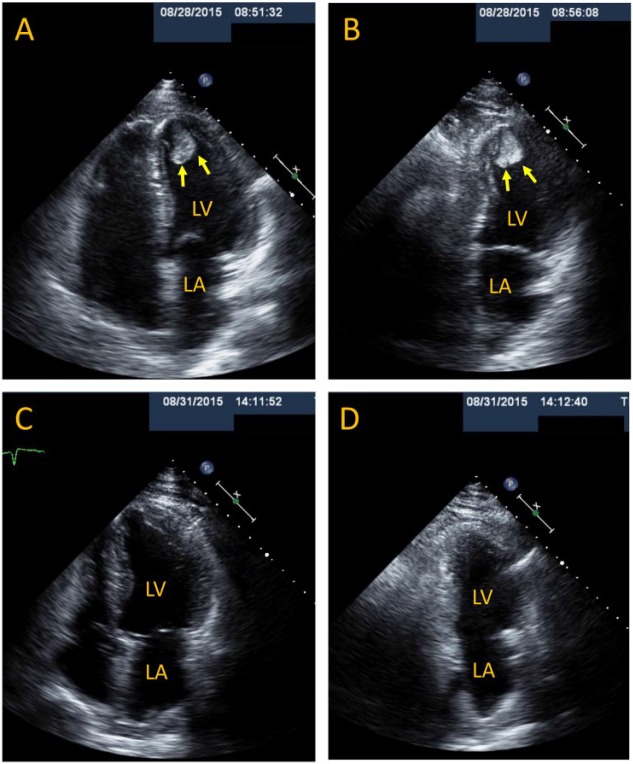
Resolution of a large apical LV thrombus within 3 days of anticoagulation. (A, B) Two-dimensional apical 4-chamber (A) and 2-chamber (B) TTE views demonstrate a large (2.4 × 2 cm), elongated, with a narrow attachment, mobile, and heterogeneously echoreflectant apical LV thrombus (arrows). Repeat 4-chamber (C) and 2-chamber (D) TTE views 3 days after initiation of intravenous unfractionated heparin demonstrate complete resolution of the apical LV thrombus. Abbreviations: LA, left atrium; LV, left ventricle; TTE, transthoracic echocardiography.
